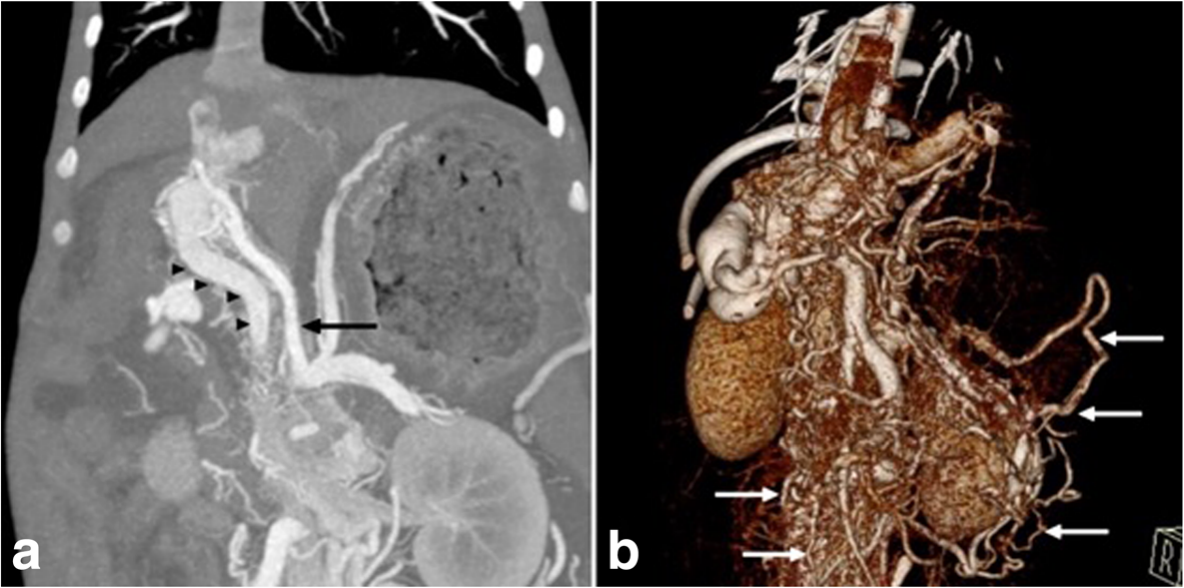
Preprocedural imaging in patient 2. a) CT angiography demonstrates a large hepatic AVM with arterial inflow arising from an enlarged celiac artery (black arrow) with early shunting into an enlarged and arterialised portal vein (arrow heads). b) 3D volumetric reconstruction shows innumerable portosystemic collaterals (white arrows)
